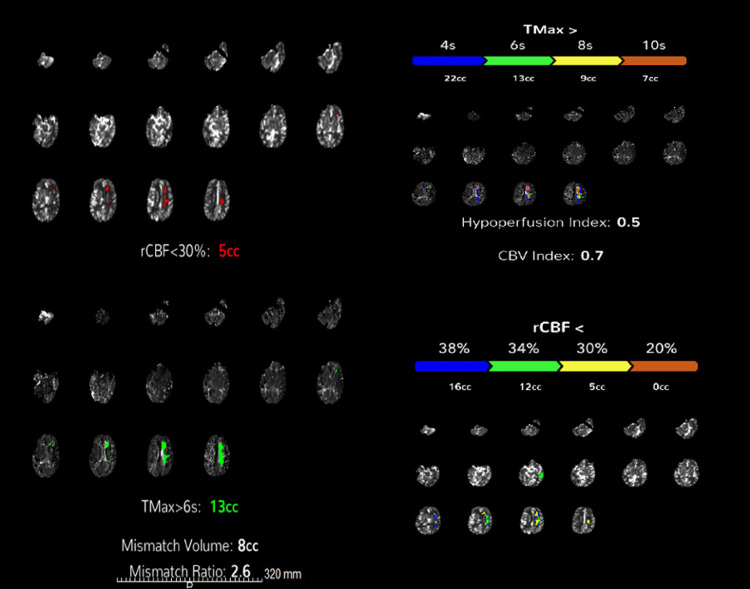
CT perfusion (CTP) imaging demonstrating ischemic core and penumbra. Multimodal CTP maps demonstrate perfusion abnormalities in the left ACA territory. The relative cerebral blood flow (rCBF <30%) map shows a small infarct core of 5 cc. The Tmax >6s map reveals a larger area of hypoperfusion measuring 13 cc, resulting in a mismatch volume of 8 cc and a mismatch ratio of 2.6. The hypoperfusion index is 0.5, and the CBV index is 0.7, suggesting relatively preserved collateral status and a favorable perfusion profile. ACA: anterior cerebral artery; CBV: cerebral blood volume
